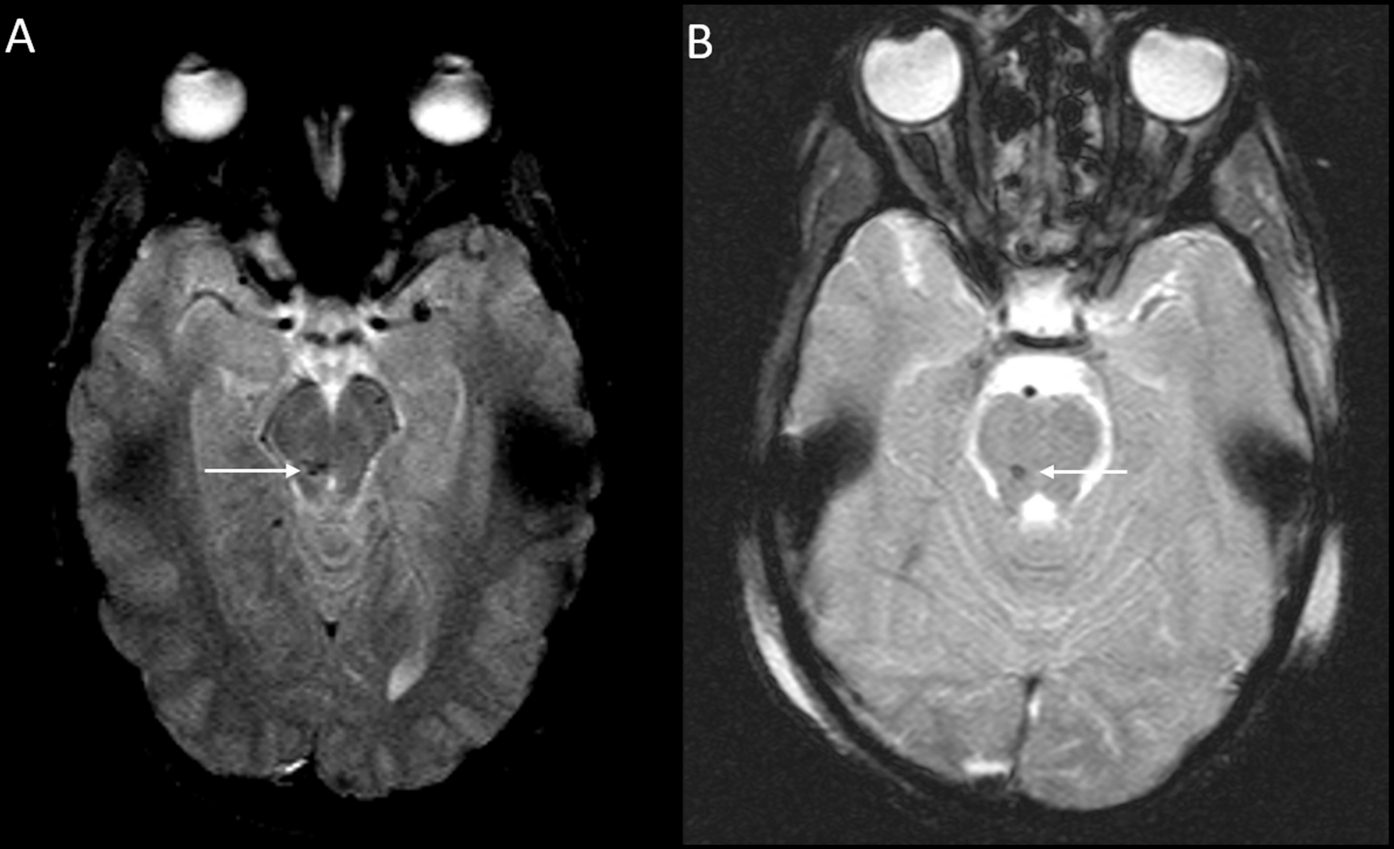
DAI Zone 3 (Posterior fossa) MRI Findings. (A) axial T2^*^ weighted image showing susceptibility effect in the right midbrain, and (B) axial T2^*^ weighted image in a different severe pediatric TBI patient showing susceptibility effect in the right pons.
